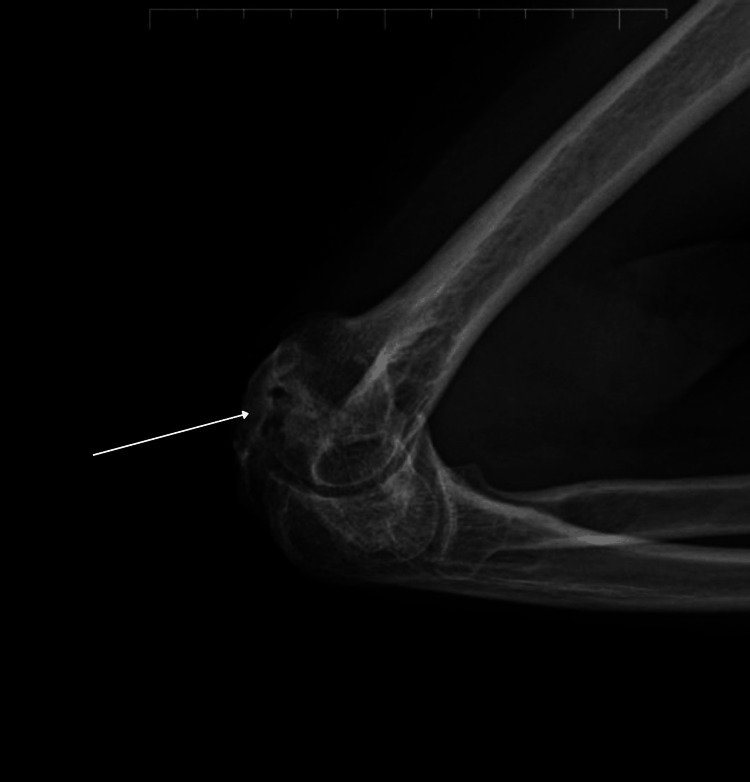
Pre-operative X-rays show ankylosis of elbow joint with bridging bone olecranon and distal humerus antero-posterior (AP) view
